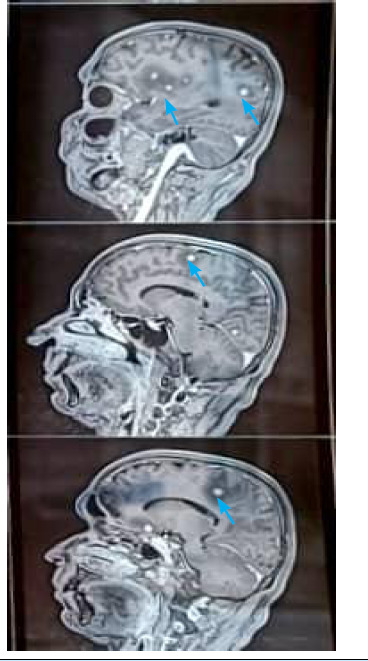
MRI brain (T1, sagittal view) showing multiple ring-enhancing lesions.
